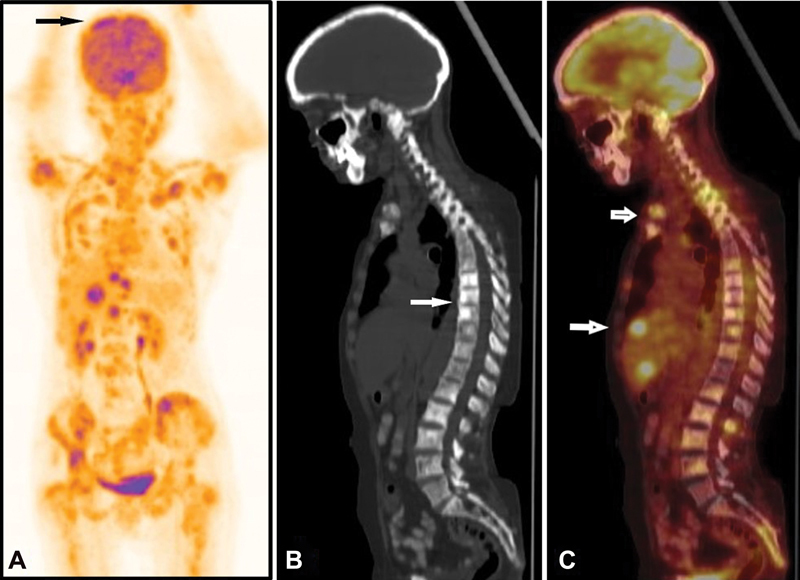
F-18 FDG PET CT maximum intensity projection (MIP) image (
